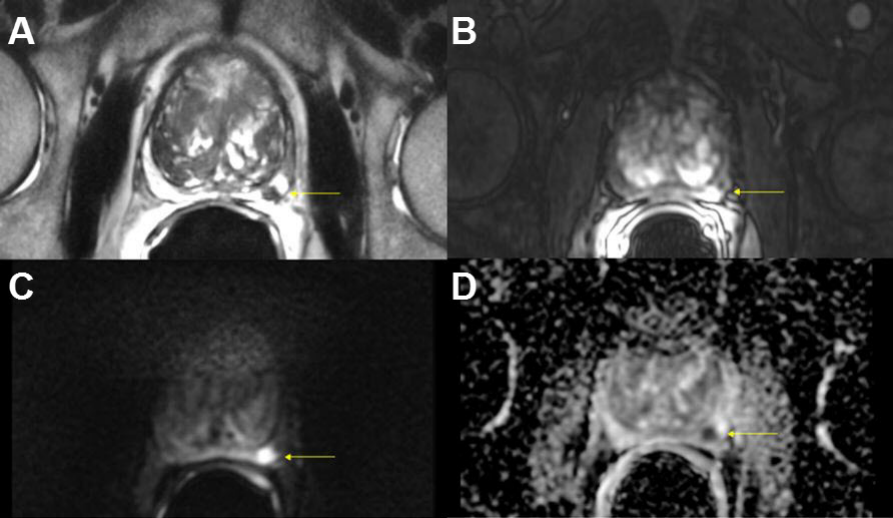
Multiparametric MRI of a Typical Malignant Prostate Nodule in a 61-Year-Old Male Left peripheral zone lesion showing low signal on T2-weighted images (A), early avid enhancement on T1-weighted images after gadolinium injection (B), high signal on b=1400 ms diffusion-weighted images (C), and low signal on ADC map (D).
